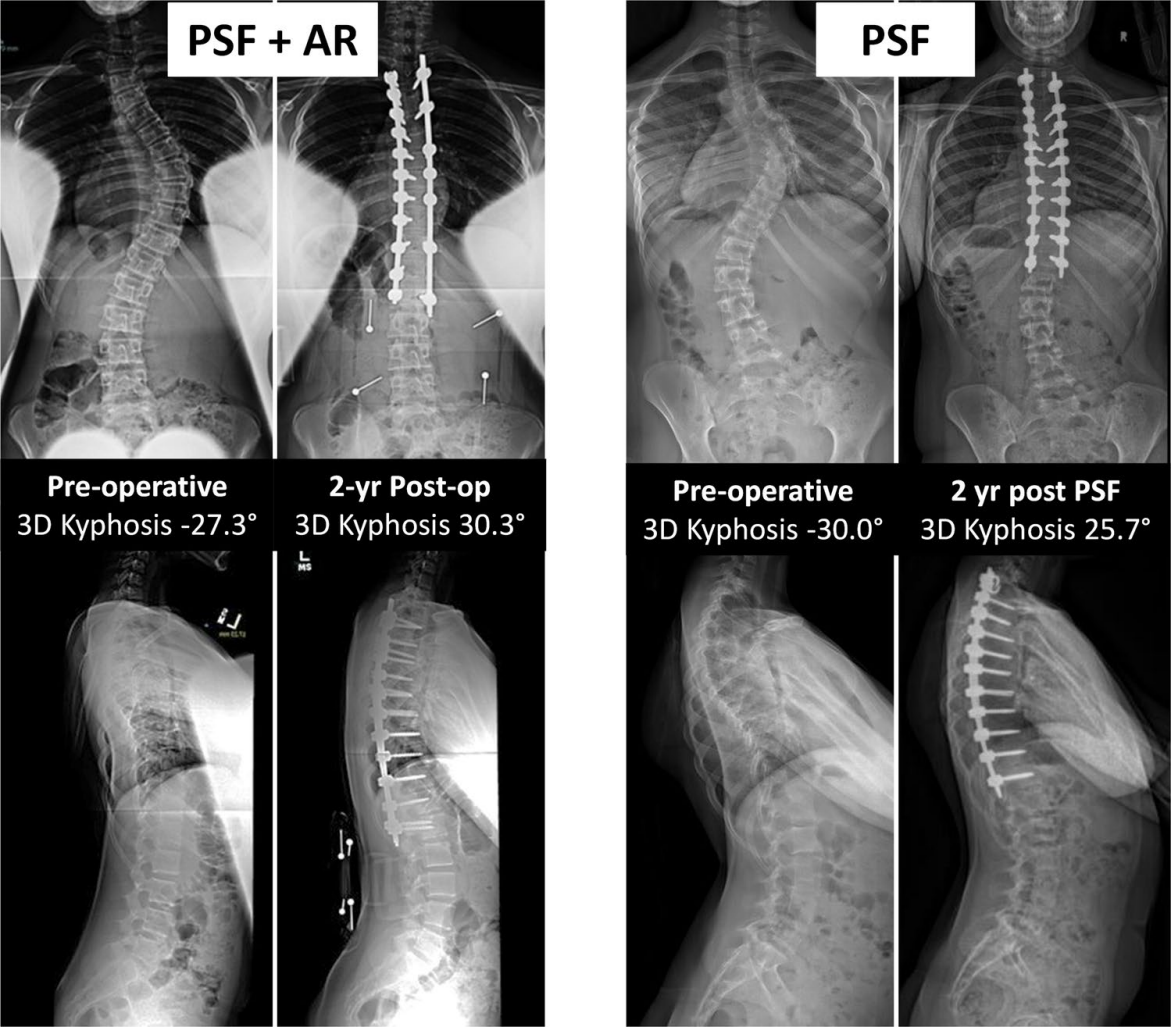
Pre- and post-operative radiographs of representative patients from each study cohort. Both cases demonstrate pre-operative thoracic lordosis, which was successfully corrected with Posterior Spine Fusion with Anterior Release (PSF + AR) or Posterior Spine Fusion alone (PSF)
